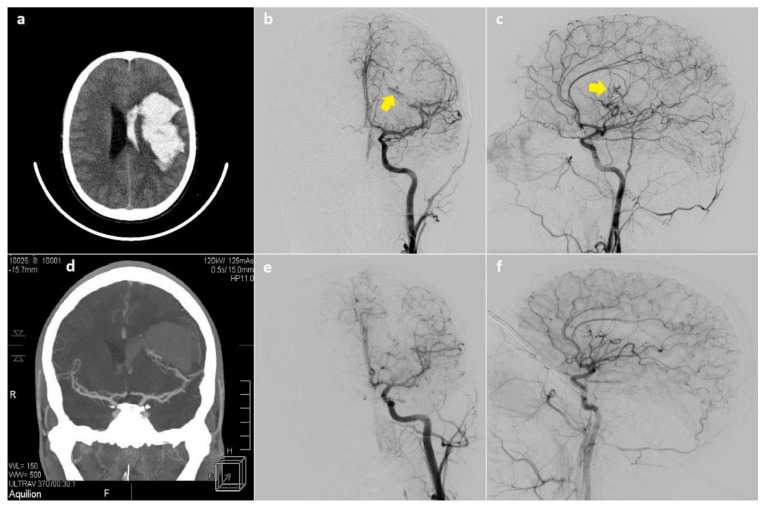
Imaging studies of the 16-year-old male patient. CT scan revealed intracerebral and intraventricular hemorrhage in the left hemisphere (a,d). Preoperative angiography demonstrated Spetzler-Martin III, arteriovenous malformation in the left temporal lobe (yellow arrows) (b,c). DSA examination performed 1.5 years after surgery showed complete excision of the malformation (e,f).
